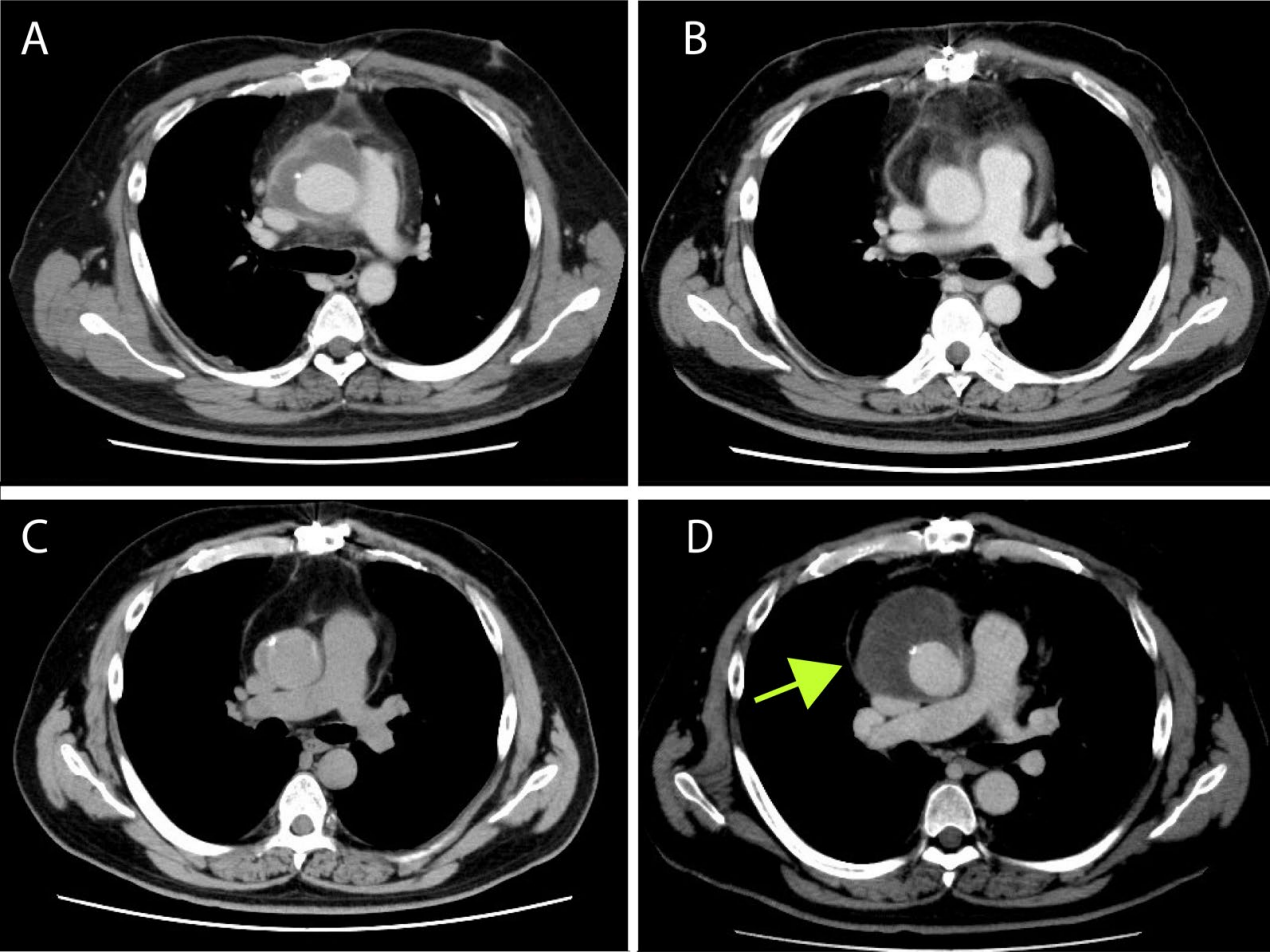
Findings on CT scans during the first 5 years after the initial surgery. a POY 1. Residual fluid was detected around the ascending aortic graft. An infected graft was diagnosed based on a positive blood culture and laboratory test results. b POY 1. A CT scan obtained after omentoplasty. c POY 3. A CT scan showing that the mediastinum was not filled. d POY 4 Residual fluid was seen in the anterior mediastinum around the ascending aortic graft (green arrow). CT, computed tomography; POY, postoperative year
